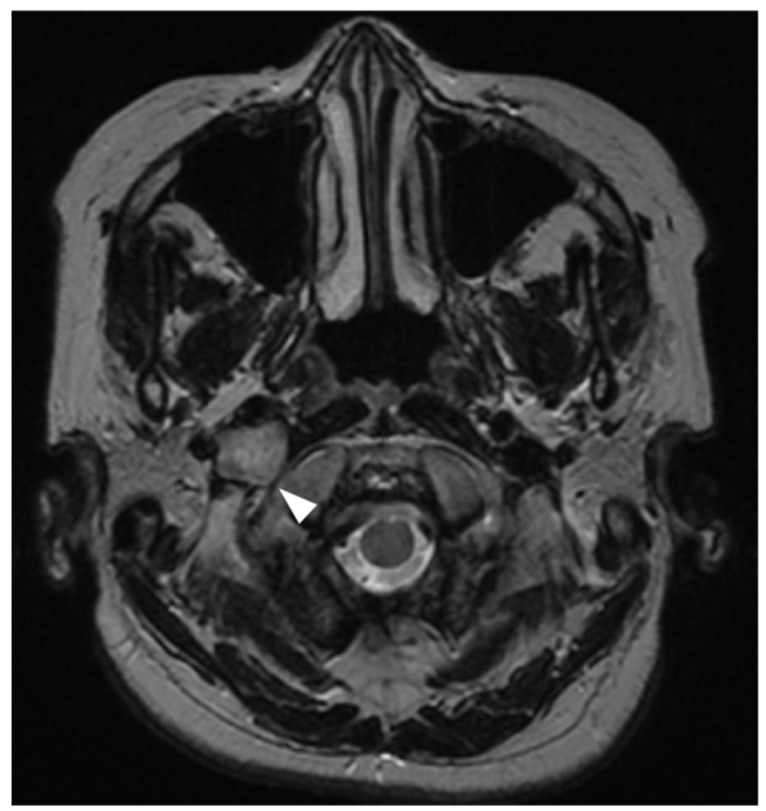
Axial T2-weighted magnetic resonance imaging demonstrating a 17 mm mass lesion located dorsal to the right internal carotid artery (arrow head).
